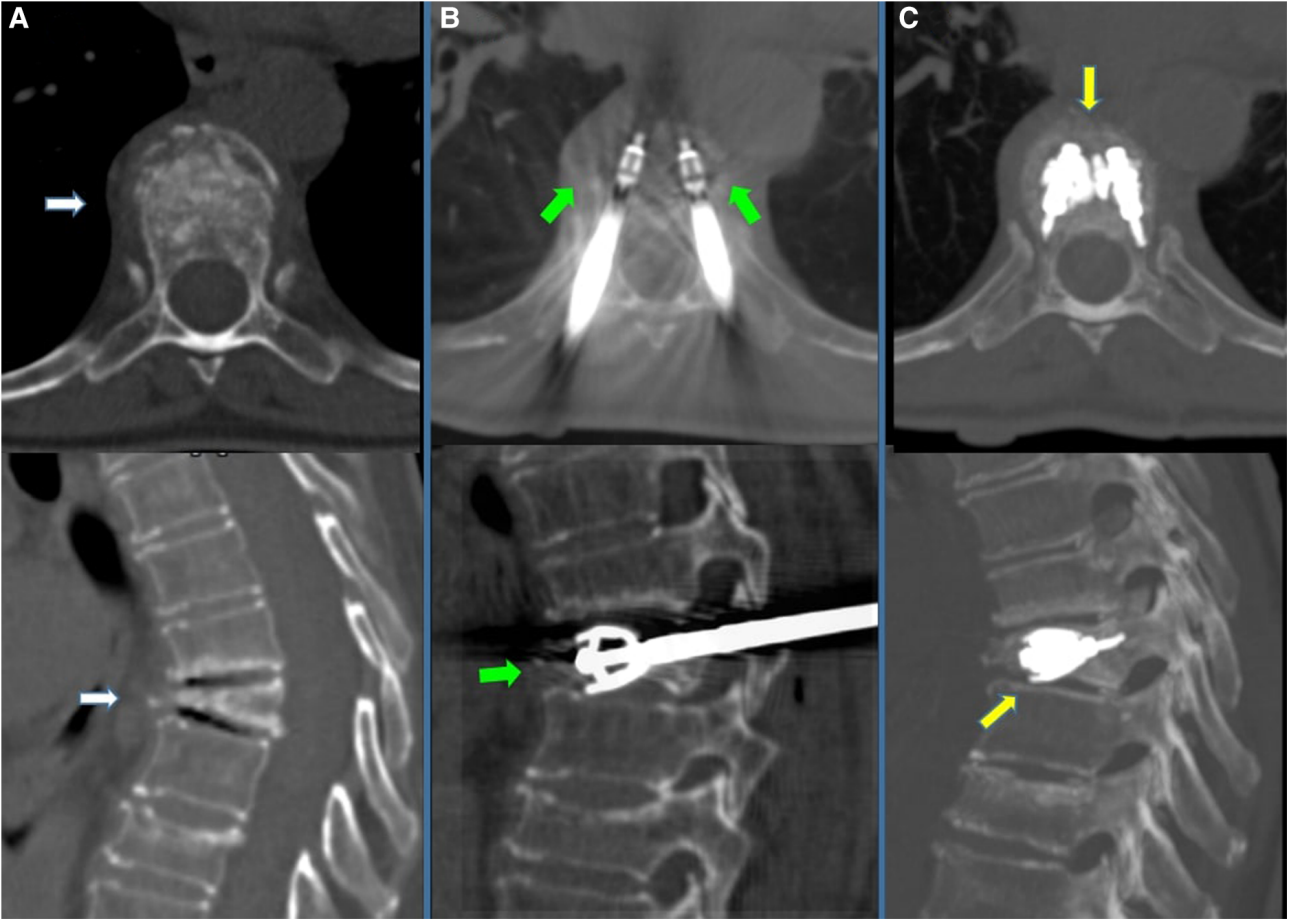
70-year-old female with a diagnosed MM with dorsal pain. (A) CT scan in axial and sagittal planes showing T6 vertebral compression fracture, with significant reduction in the height of the anterior wall (white arrows). (B) CT scan in axial and sagittal planes; after preparation of both sides, two SpineJack® implants were inserted into the vertebral body and were gradually and simultaneously deployed (green arrows). (C) CT scan using MIP (Maximum Intensity projection) reconstruction in axial and sagittal planes; Post-procedure control images showed a correct expansion of the vertebra with a homogeneous distribution of the vertebral cementum. No cement leaks or complications.
